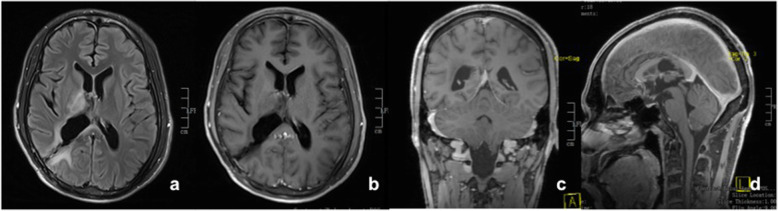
a Axial T2 FLAIR and b–d axial, coronal, and sagittal sections on T1 contrast MRI of brain showing altered signal involving right thalamus, posterior limb of internal capsule (reduced compared to pre-radiotherapy scans) with no abnormal enhancement in this region. Subtle persistent signal alteration in the medial left thalamus. Mild persistent focal leptomeningeal enhancement seen in right side of superior cerebellar and quadrigeminal plate cistern. No residual enhancing mass lesion in the right lateral ventricle or corpus callosum
